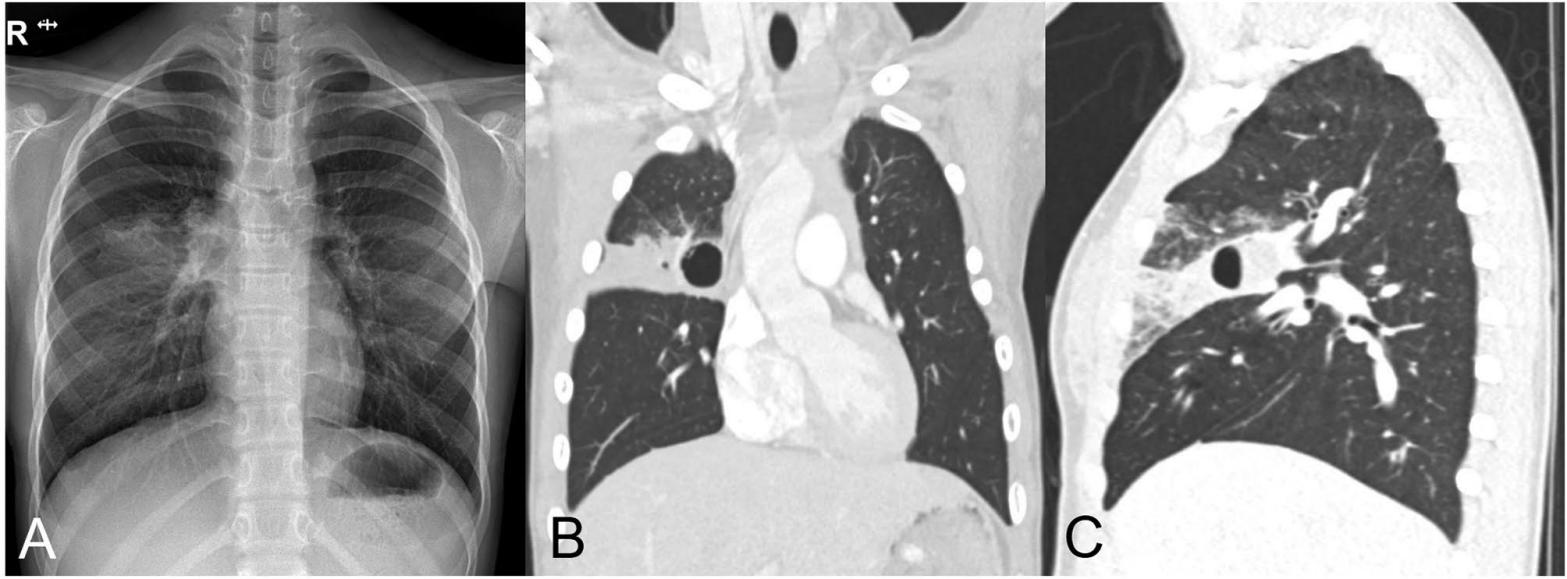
Preoperative imaging studies: (A) chest X-ray demonstrating cystic lesion and consolidation in right hilar area and (B, C) computed tomography of chest (coronal and sagittal views) with similar findings confined to anterior segment
